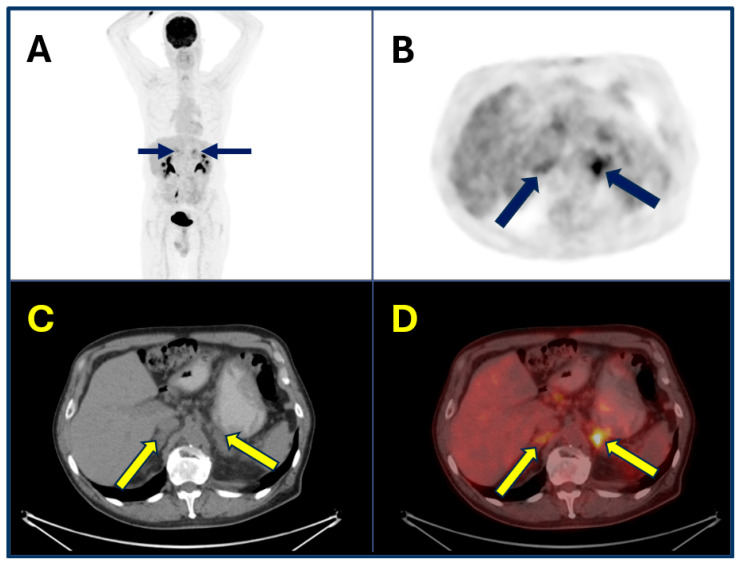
A 49-year-old man with metastasized melanoma received immunotherapy with ipilimumab and nivolumab. Six months after therapy initiation, FDG-PET/CT [(A,B), (A): maximum intensity projection; (B): axial attenuation corrected section; (C,D), CT and fused PET/CT axial images] revealed slightly enlarged and ^18^F-FDG-avid adrenal glands ((A,B): blue arrows; (C,D): yellow arrows). Subsequent ACTH stimulation testing confirmed adrenal insufficiency, although the patient remained asymptomatic, allowing timely hormone substitution to prevent overt adrenal insufficiency.
